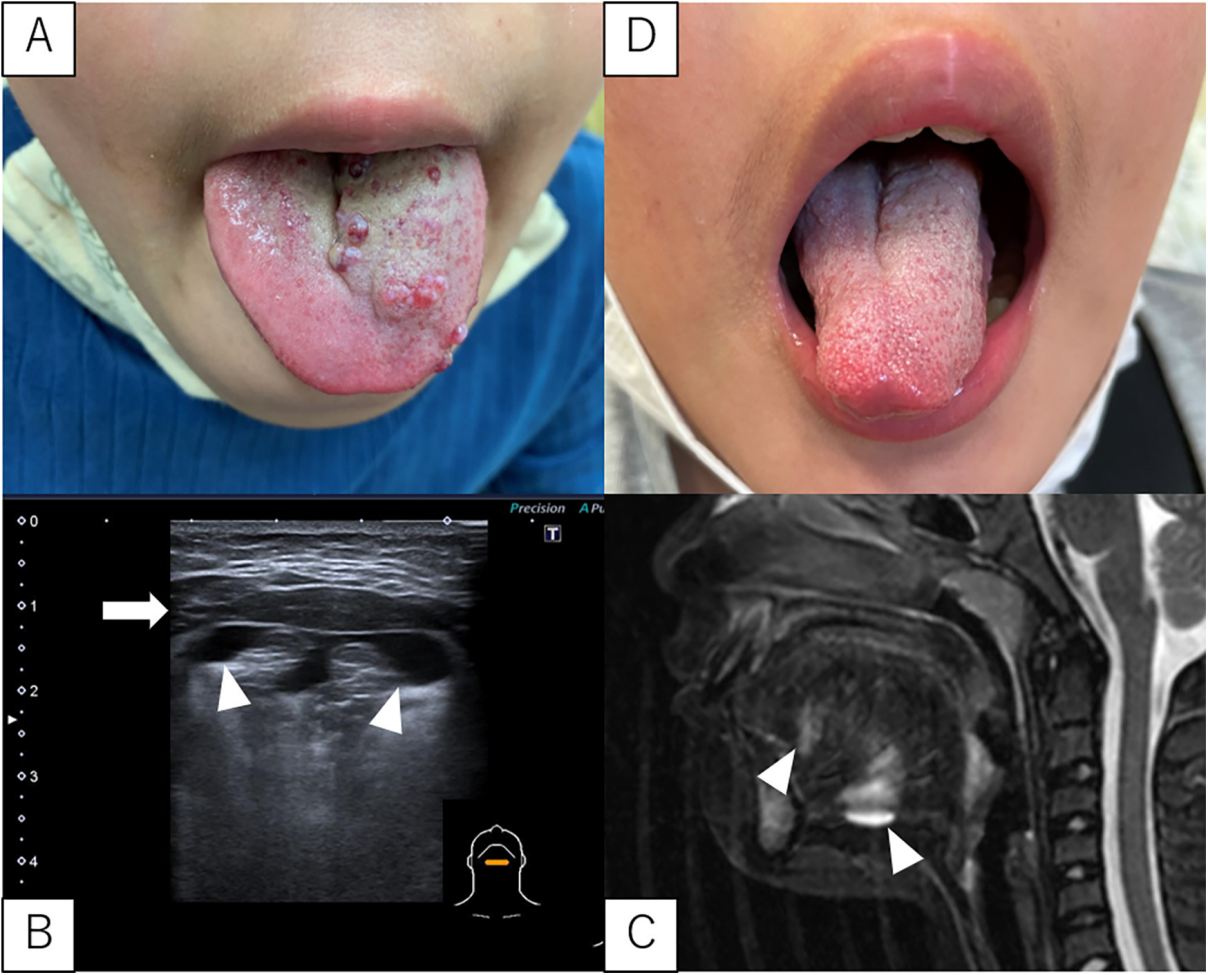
SLM and LM in case 4. (A) Pre-sclerotherapy gross appearance of the SLM on the tongue. (B,C) Ultrasonography (from below the mandible) and MRI (T2, sagittal section) showing a deep LM in the mylohyoid muscle and tongue. White arrowheads indicate the LM. The white arrows indicate the mylohyoid muscle. (D) Gross appearance after sclerotherapy SLM are almost unidentifiable. LM, lymphatic malformations; SLM, superficial lymphatic malformation; MRI, magnetic resonance imaging.
